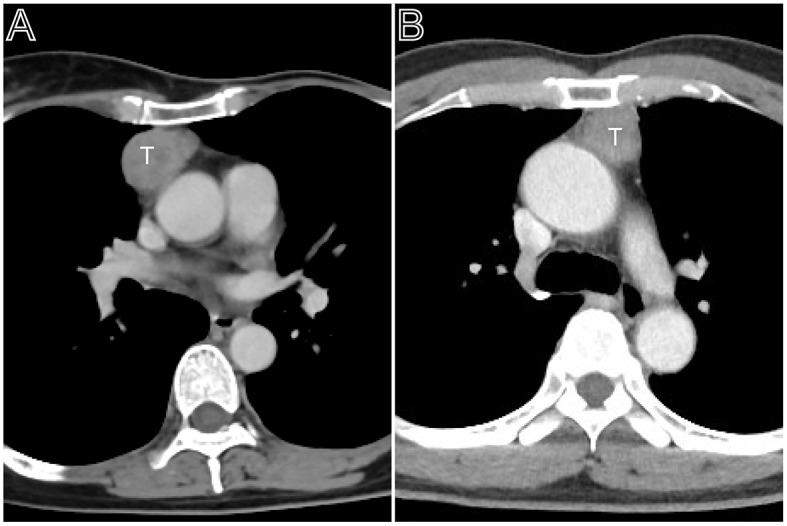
Masaoka–Koga stage II thymomas. (A) Axial contrast-enhanced chest CT image showed a thymoma with a clear contour (Masaoka–Koga stage IIa and WHO classification B1), which was first found at the time of physical examination in a 55-year-old woman. (B) A 50-year-old woman mainly complains of general fatigue and subsequently was confirmed myasthenia gravis. Axial contrast-enhanced chest CT image obtained at the level of the left pulmonary trunk showed a thymoma with a slight lobulated contour (Masaoka–Koga stage IIb and WHO classification AB).
